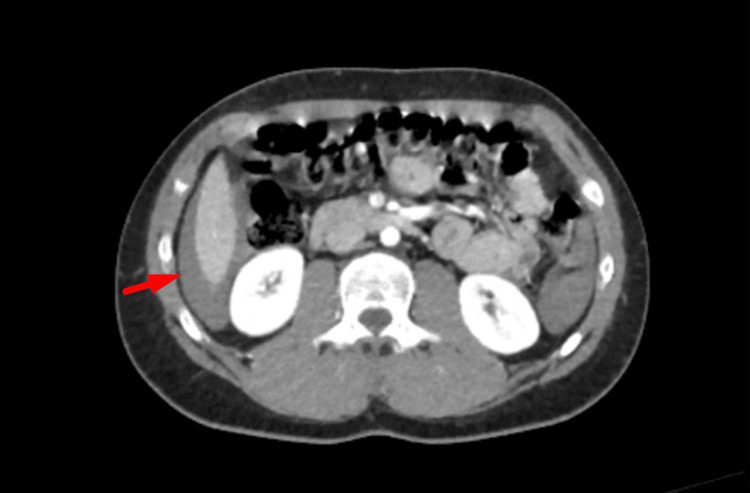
CT cross-section demonstrating haemoperitoneum CT: computed tomography
